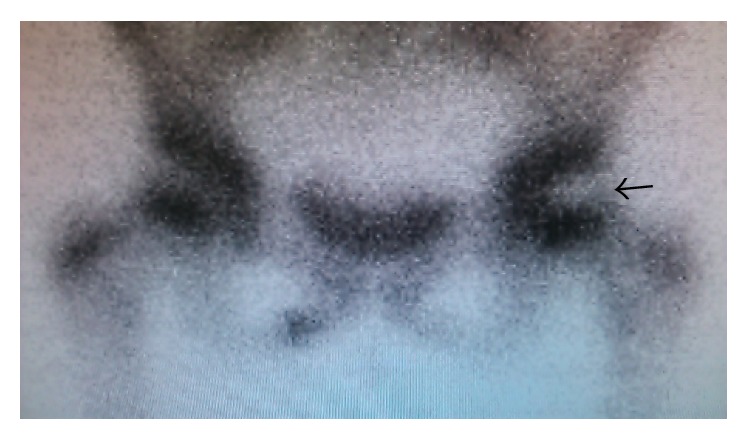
Bone scintigraphy findings. A cold-in-hot pattern is shown at the left femoral capital epiphysis (arrow).
